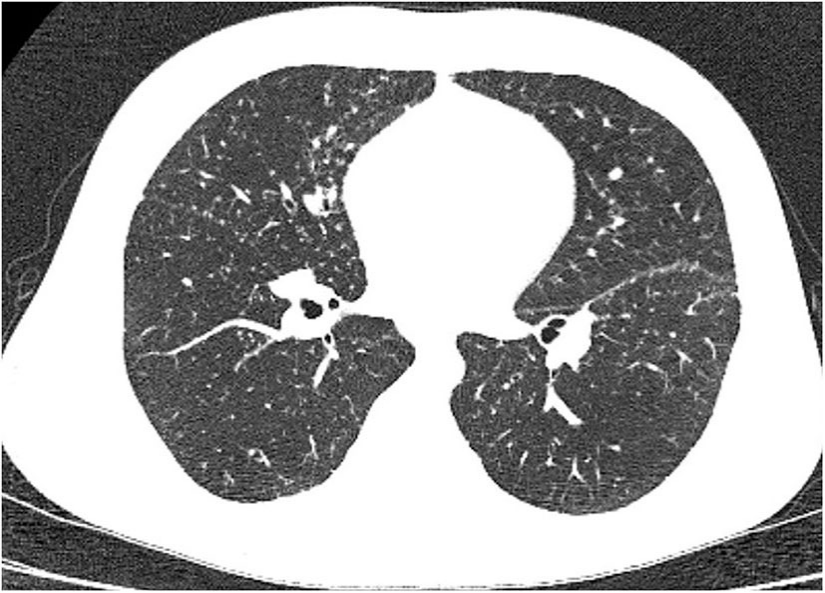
Lung high-resolution computed tomography: multiple small pulmonary nodules with perilymphatic distribution (clearly seen along pleural fissures).
